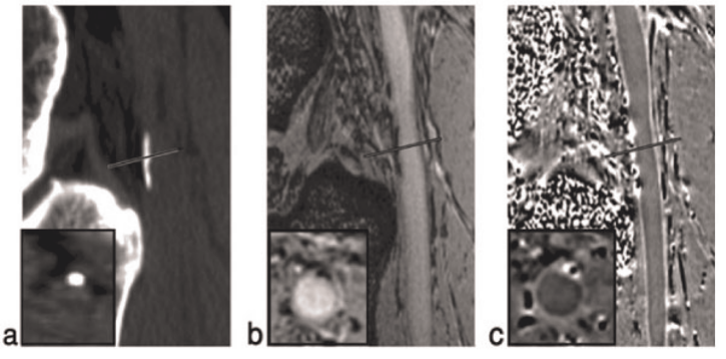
(a) CT scan showing calcification at the edge of the popliteal artery just behind the knee. (b) Magniture gradient echo image showing the signal loss from the calcification of the same area. (c) Phase image showing the diamagnetic effect from the calcification. Note the simliar shape and extent of the calcification in both the CT and MR results. Inserts are zoomed images of the cross-section of the vessels in reformatted transverse images.
